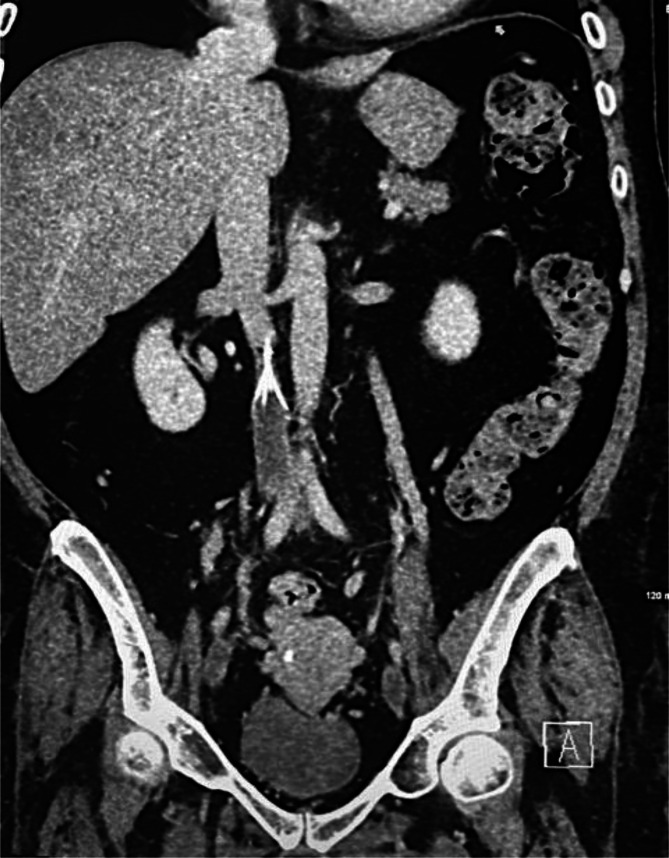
Coronal CT image demonstrates IVC thrombus below the filter. IVC, inferior
